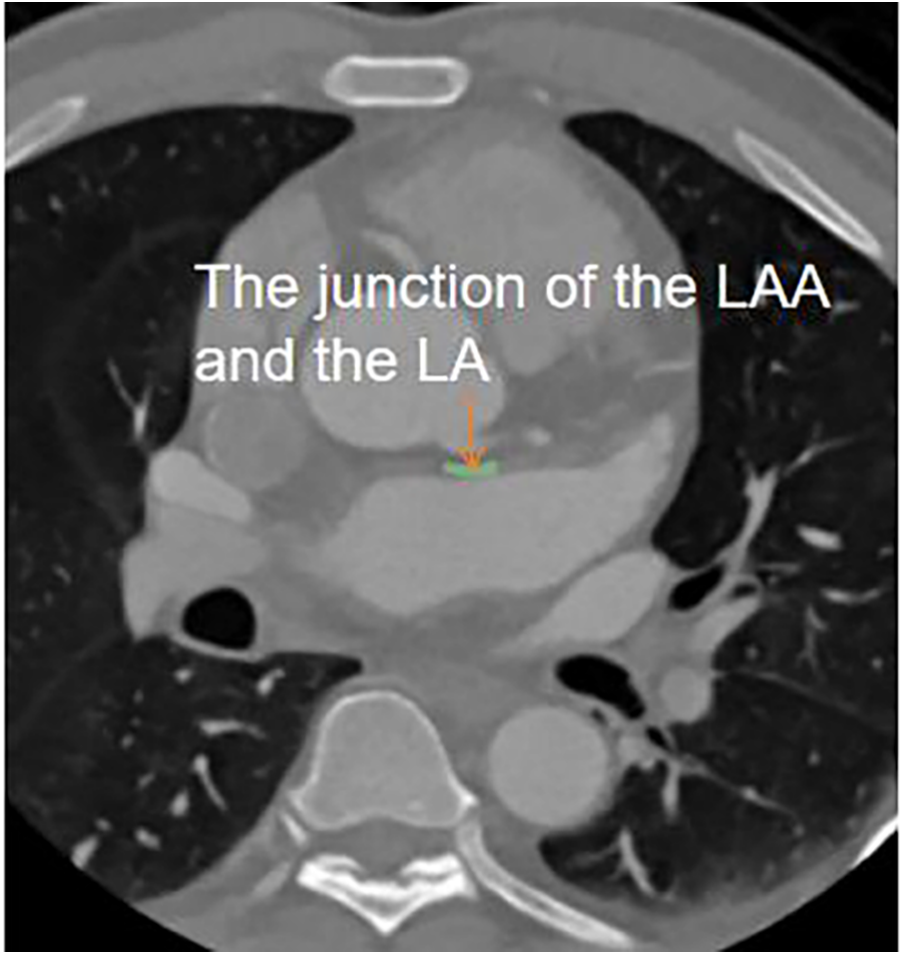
ROI delineation. On the axial image of cardiac CT, the myocardium at the junction of the LAA and the LA is delineated (green). The length of ROI was 1 cm in total.
